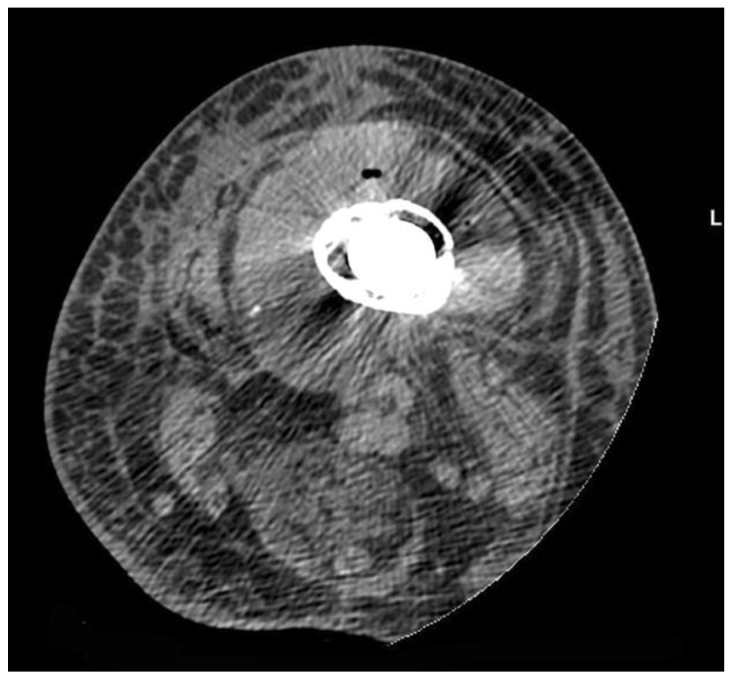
Computed tomography (CT) features: axial scan showing a fluid collection with increasd density surrounding infected bone with a prosthetic implant. Note the swelling and hyperdensity of soft tissues due to edema.
